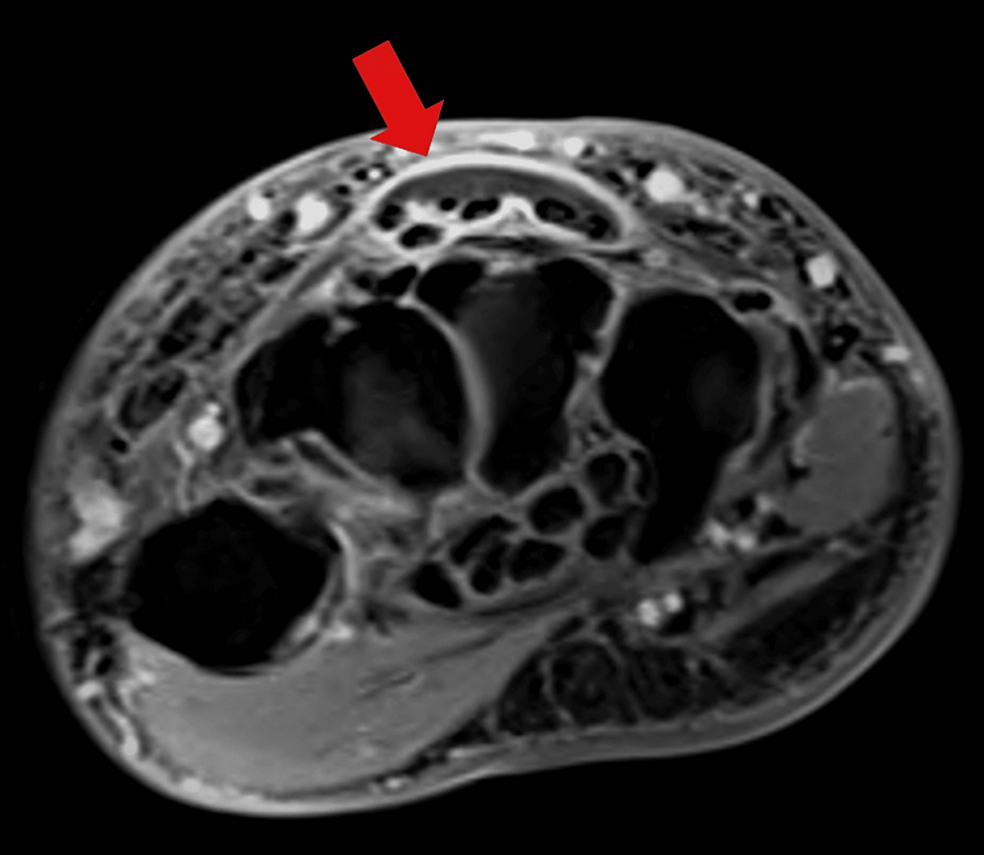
MRI axial T1 image post-contrast showing enhancement of and fluid within tendon sheaths
